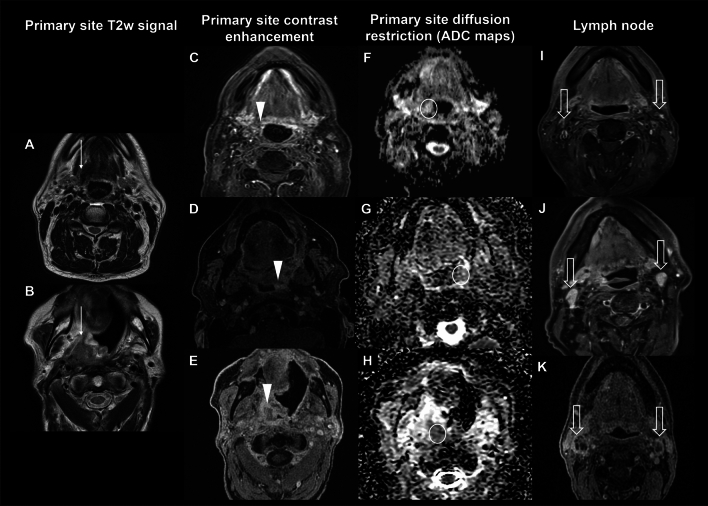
Examples of oropharyngeal carcinoma features after treatment. A Marked hypointene soft tissue and linear submucosal hyperintensity in the right tonsil (arrow), representing scar and edema. B Intermediate signal intensity (“evil grey”) soft tissue in the right tonsil (arrow), representing tumor recurrence. C Thin linear mucosal enhancement in the right tonsil (arrowhead), representing inflammation. D Focal non-mass-like mucosal enhancement in the left tonsil (arrowhead). E Mass-like enhancement in the right tonsil, representing tumor recurrence (arrowhead). F Absence of diffusion restriction (hyperintensity in ADC map) in the right tonsil (circle), representing edema. G Focal reduced diffusion (hypointense in ADC map) without a mass-like soft tissue in the left tonsil (circle). H Reduced diffusion (hypointense in ADC map) within a mass-like soft tissue in the right tonsil (circle), representing tumor recurrence. I Hypoenhancing nodal tissue without irregular borders (empty arrows) in a patient with base of the tongue carcinoma. J Residual nodal tissue with heterogeneous enhancement (empty arrows) in a patient with base of the tongue carcinoma. K Enlarging lymph node with irregular borders and new necrosis (empty arrows) in a patient with soft palate carcinoma. Some images are taken from the same patient exams (A, C, F; B, E, H; D,G; I, J). T2w, T2-weighted; ADC, apparent diffusion coefficient
